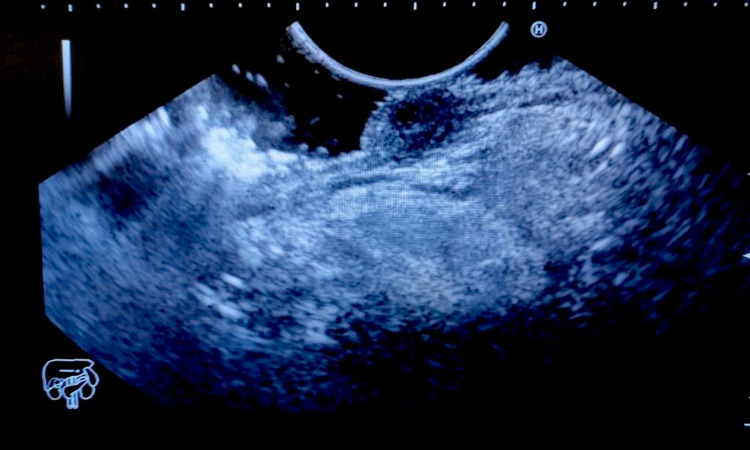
EUS showing a 2.2 cm hyperechoic lesion arising from the second layer (submucosa) with no calcification, cystic change, or ductal structure EUS (Endoscopic Ultrasound)
